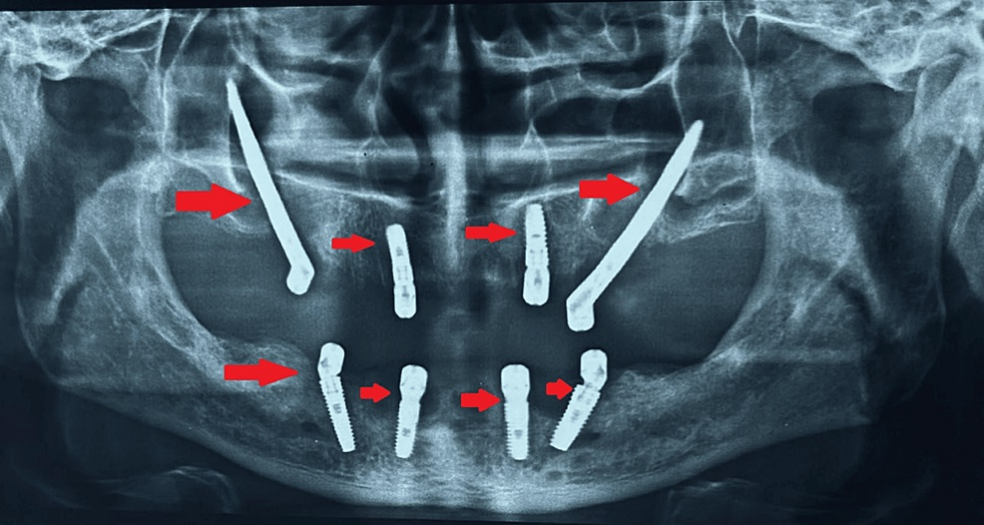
Post-implant placement radiograph Arrows are indicative of implant position.
